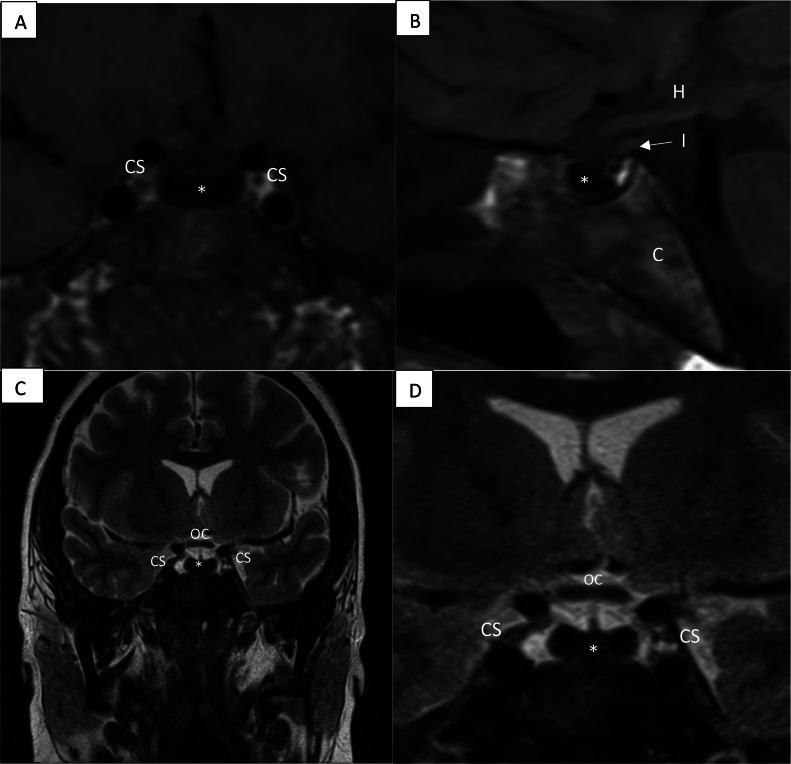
MRI of the suprasellar/pituitary region of the brain. T1 coronal (A) and sagittal (B) and T2 coronal images (C and D) with diffuse T1 and T2 signal loss within the pituitary gland (*). T1 signal of the normal pituitary gland should be isointense within the anterior segment and hyperintense in the posterior segment. For T2 sequences, the normal anterior and posterior pituitary should be isointense. The complete T1 and T2 signal loss in the pituitary is due to iron deposition from secondary hemochromatosis. The loss of signal intensity is lower than the adjacent bone signal. * = pituitary gland, CS = cavernous sinus, H = hypothalamus, I = infundibulum, OC = optic chiasm, C = clivus.
